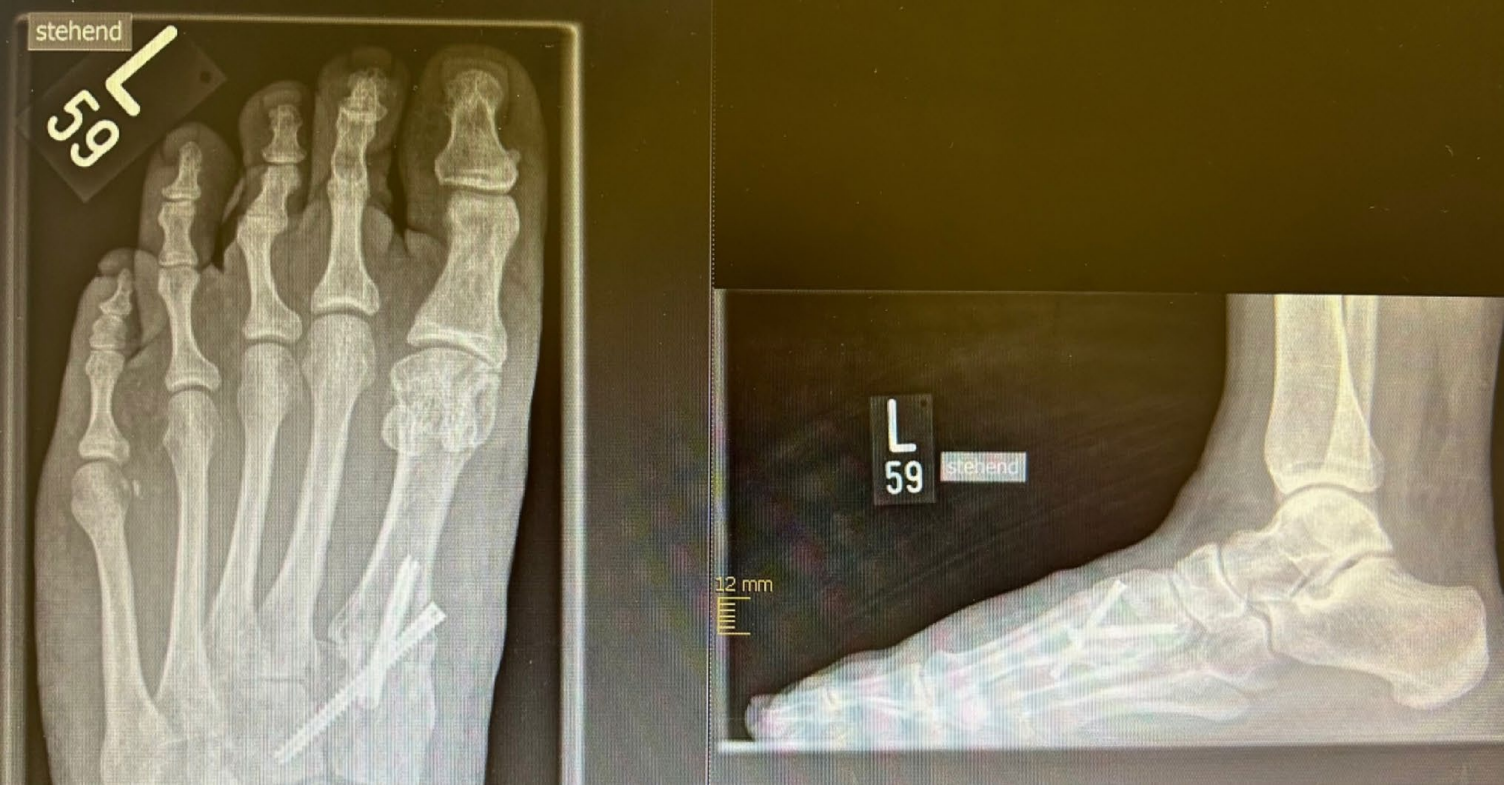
X-ray: long-term results after OS
